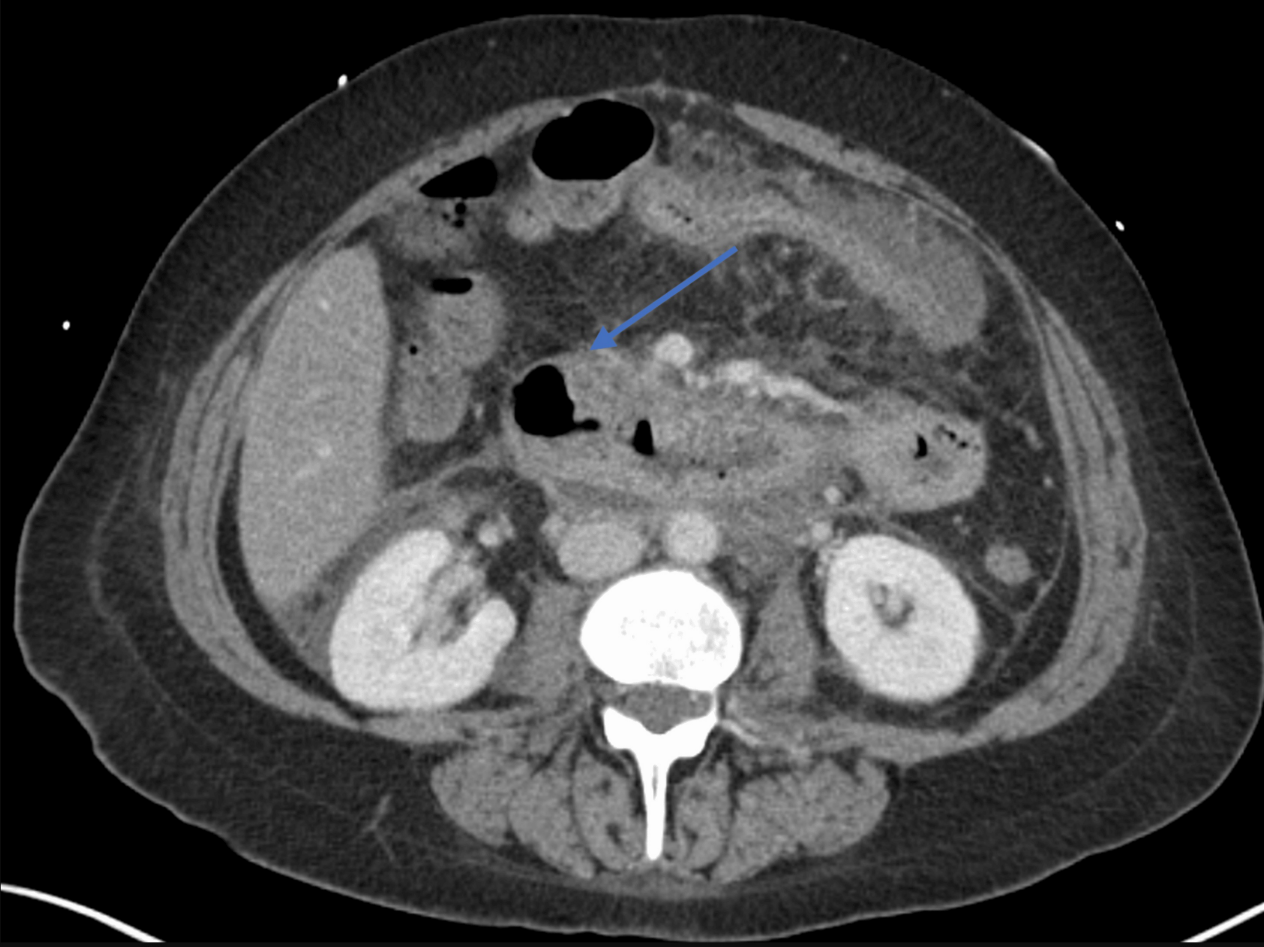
CT scan of the abdomen with IV contrast (axial view) demonstrating pancreatitis and multiple peripancreatic fluid collections consistent with severe acute pancreatitis CT: computed tomography
